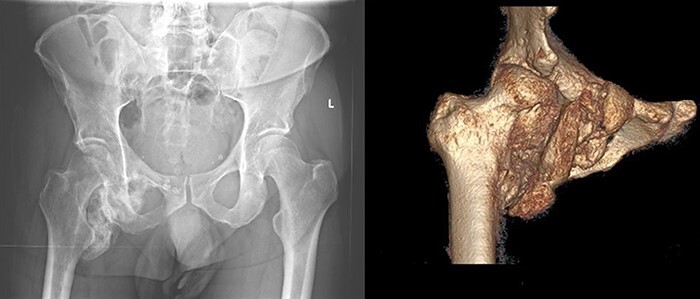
Pelvic overview radiograph and 3D reconstruction, 10 months after ICU therapy. Peripheral density of calcification in the medial/inferior area from the right hip, corresponding to HO.
